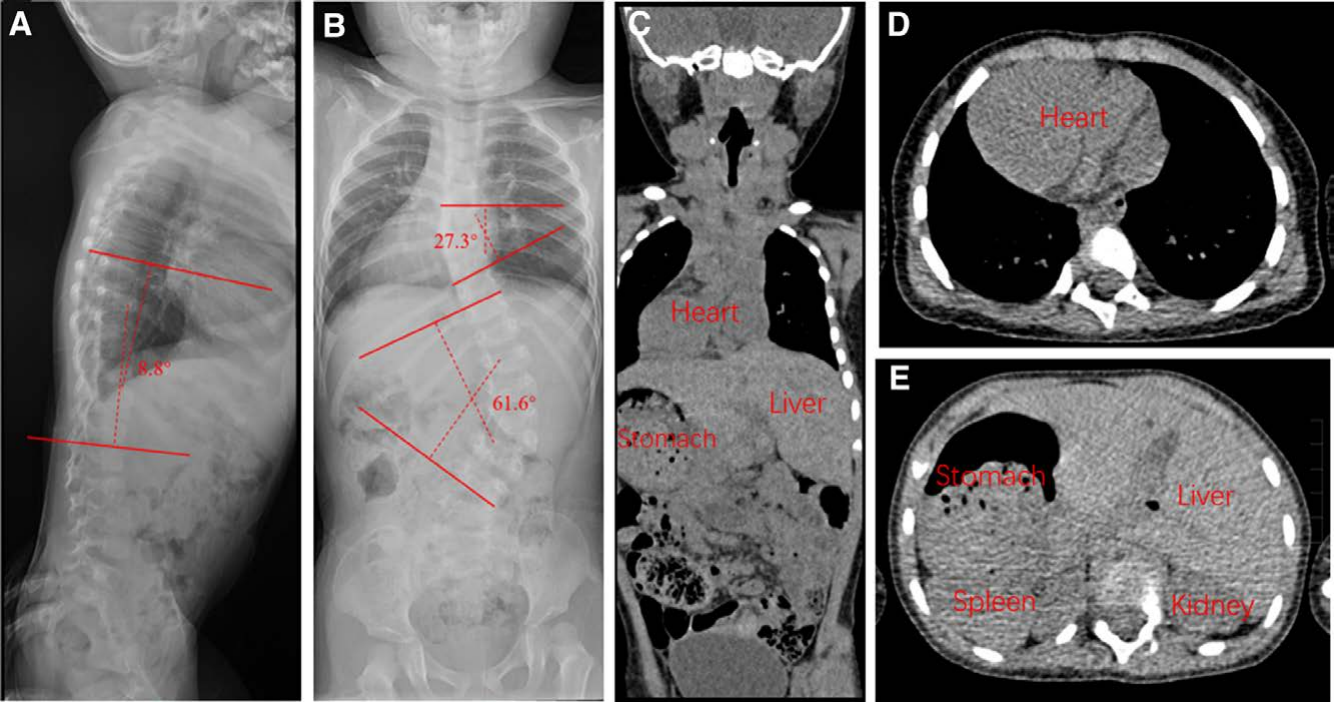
Radiographic features of the patient. (A) Lateral full spine X-ray and TK. (B) Standing full spine X-ray and Cobb angle of thoracic and lumbar curve. (C) Sagittal plane of CT. (D and E) CT of chest and abdomen. CT = computed tomography, TK = thoracic kyphosis.
